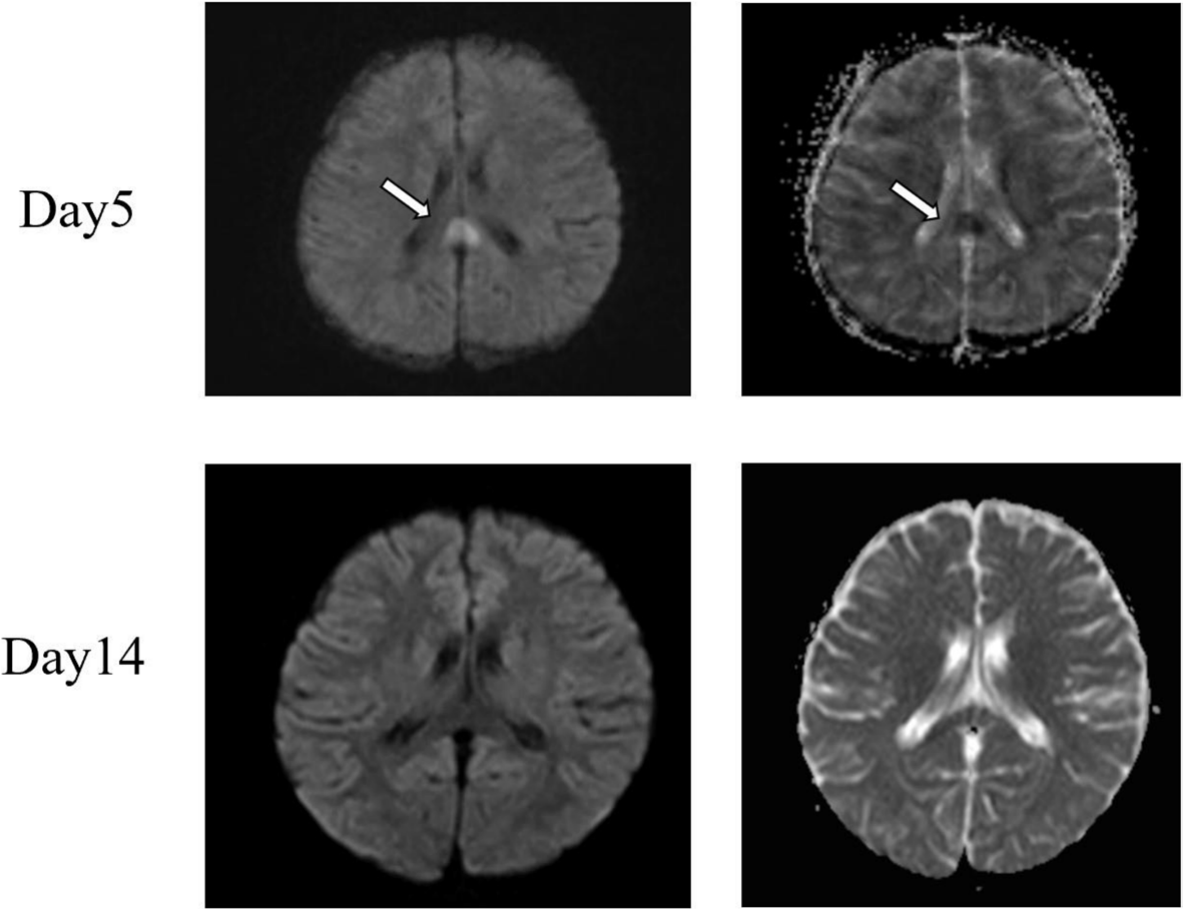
Magnetic resonance imaging (MRI) performed on day 5 and day 14. On day 5, diffusion weighted imaging of MRI shows a high signal lesion in the splenium of the corpus callosum, and apparent diffusion coefficient map shows a low signal in the same lesion. These findings disappear on day 14
